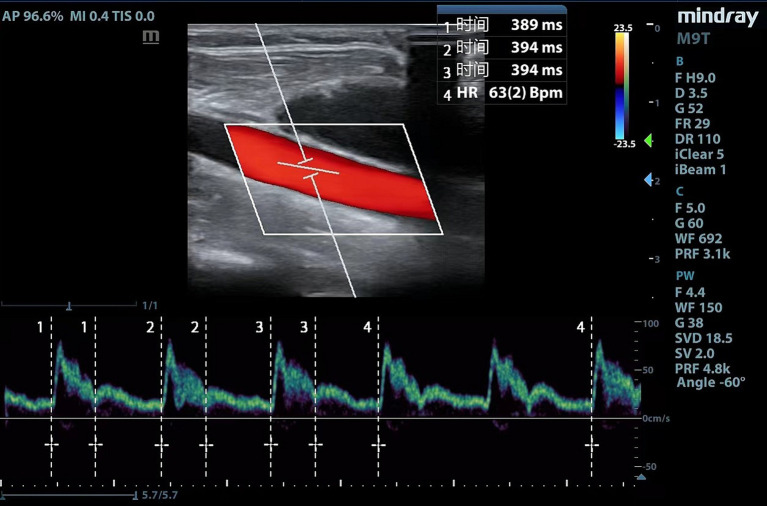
Carotid Doppler waveform.
